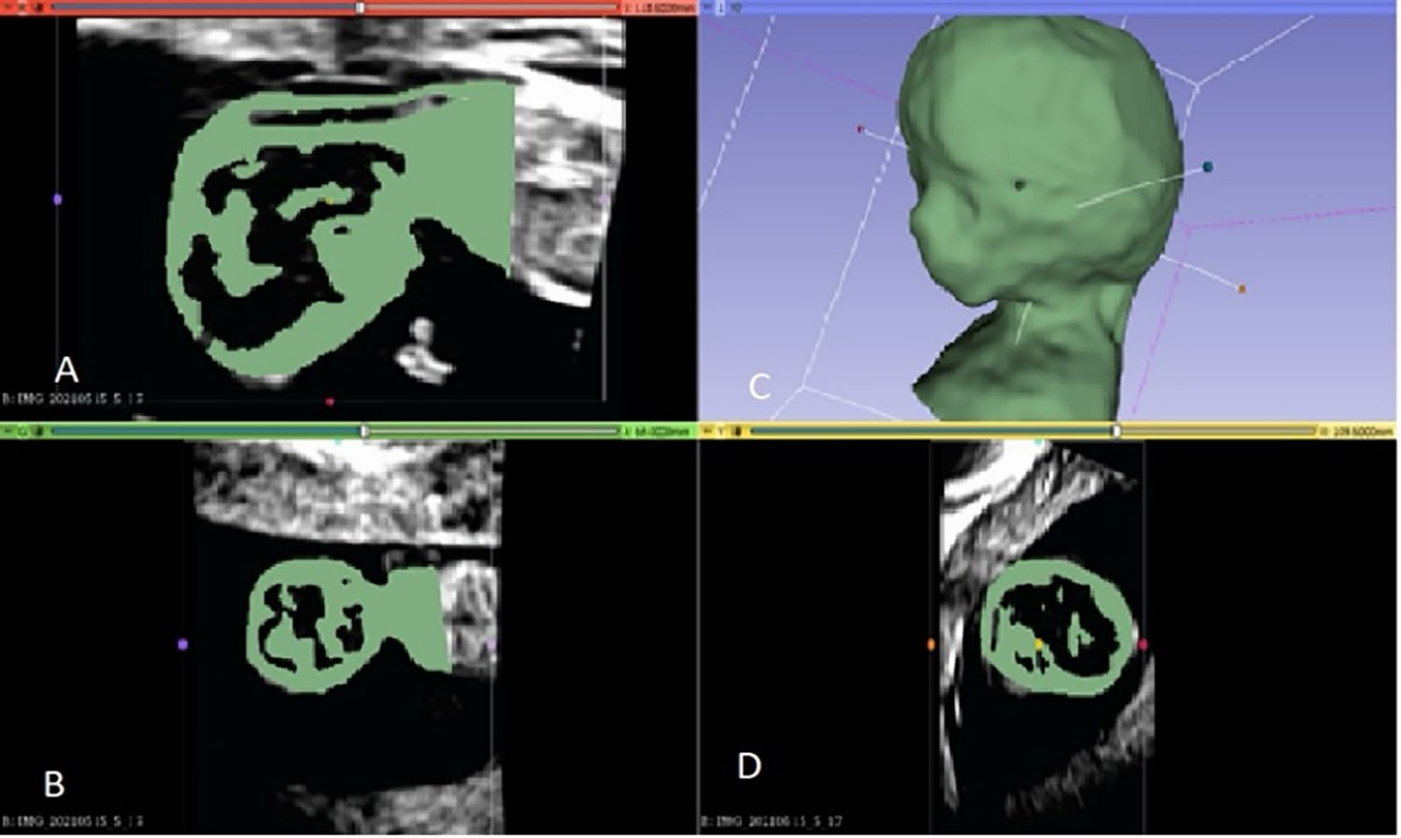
Demonstrating the reconstruction of a 3D model using ultrasound images in three different planes: A sagittal, B coronal, and D transverse. C showcases the resulting 3D model computed by the software
